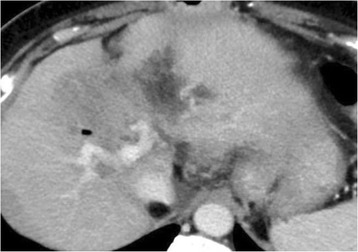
Computed tomography of the treated liver 6 years after the tumor relapse. Neither dilatation of intrahepatic bile duct nor tumor relapse is seen in the latest CT imaging
